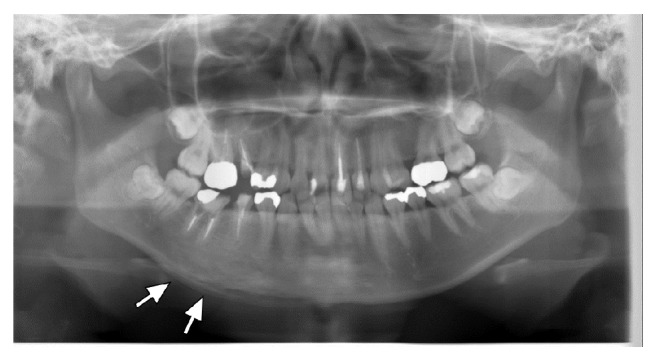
Orthopantomography. Sclerotic changes are seen in the right body of the mandible with periosteal reaction (arrows).
